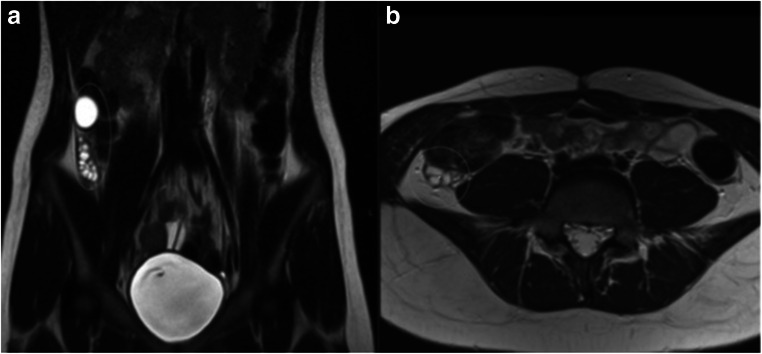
Unilateral ovarian maldescent in MRKH syndrome. T2 HASTE coronal (a) and axial (b) images depict the right ovary in an ectopic location, in the right paracolic gutter. Of note, the right ovary was reported as absent in a previous MRI examination that did not include a large FOV sequence, highlighting the importance of a dedicated MRI protocol
